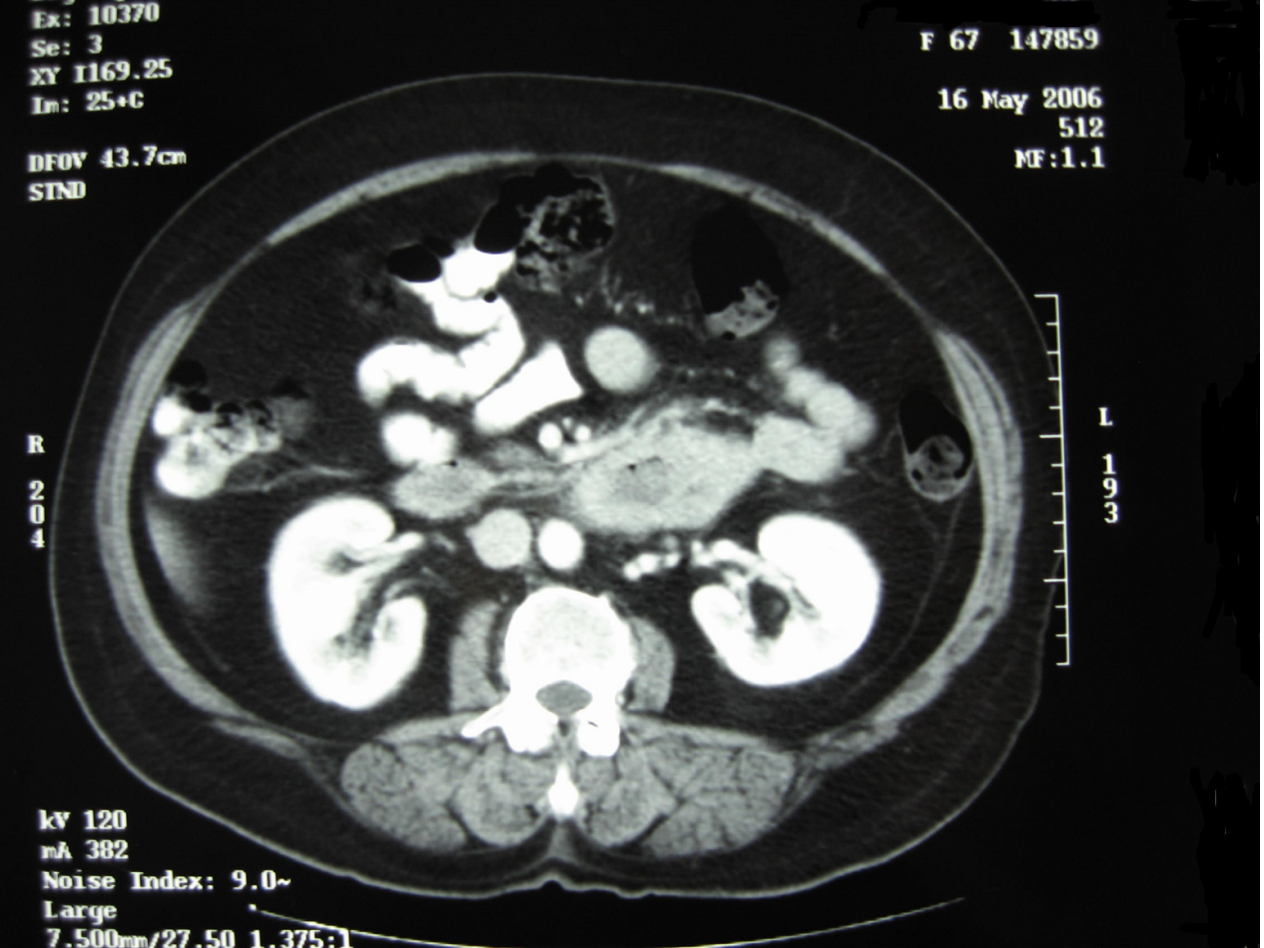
Abdominal contrast-enhanced CT scan revealing a mass in the third and fourth part of the duodenum.
